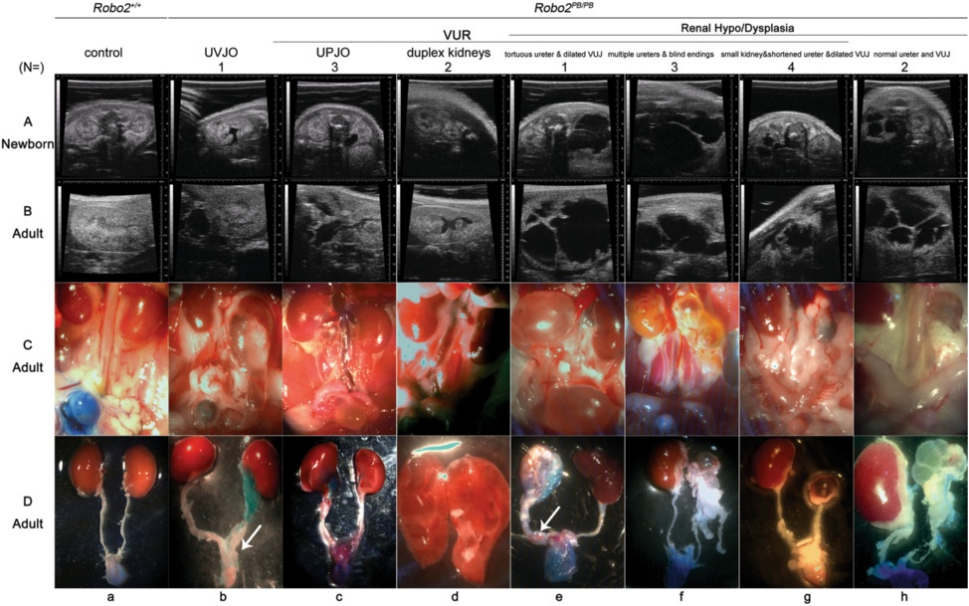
Ultrasound images and gross anatomy of urinary malformations found in *Robo2*
